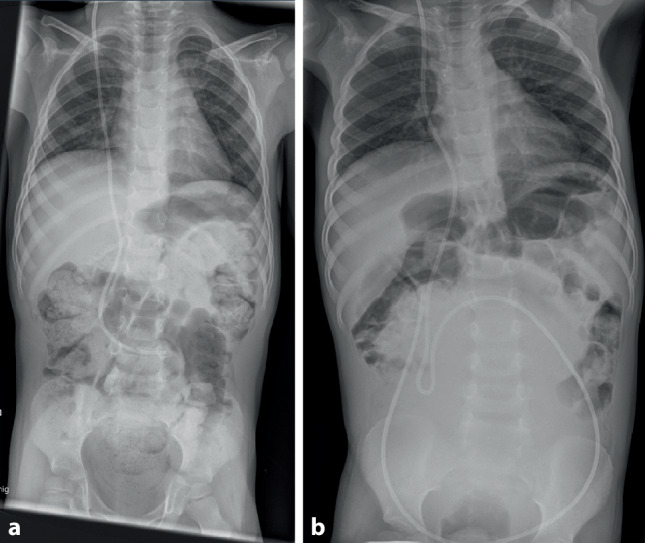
a X-ray demonstrating cranial displacement of the large intestine, b X-ray demonstrating regular intra-abdominal gas pattern
